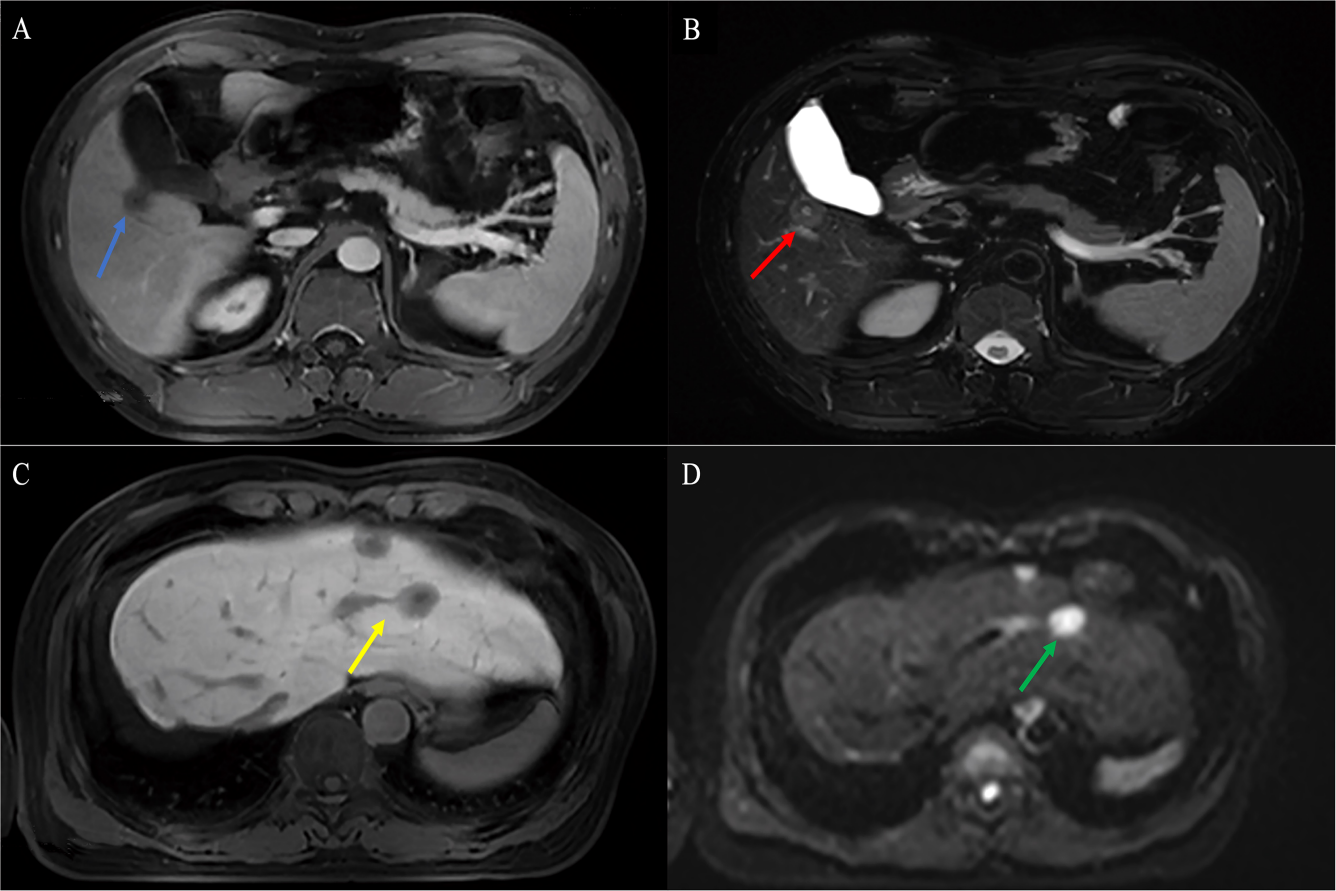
Findings of HEHE in Gd-EOB-DTPA-enhanced MRI. A 38-year-old male patient with a uninodular tumor about 1.8 cm in diameter with slight hypointensity on T1WI (blue arrow) (A) and hyperintensity in the center and outer layer, and hypointensity in the middle layer on T2WI (target sign, yellow arrow) (B). A 50-year-old female patient with well-defined nodules in segment II and IV with hypointense and clear margins on T1WI (yellow arrow) (C). Axial MRI demonstrated limited diffusion hyperintensity on diffusion-weighted imaging (DWI) (green arrow) (D). HEHE, hepatic epithelioid hemangioendothelioma; Gd-EOB-DTPA, Gadolinium ethoxybenzyl diethylenetriamine pentaacetic acid; T1WI, T1-weighted image; T2WI, T2-weighted image; DWI, diffusion-weighted imaging.
